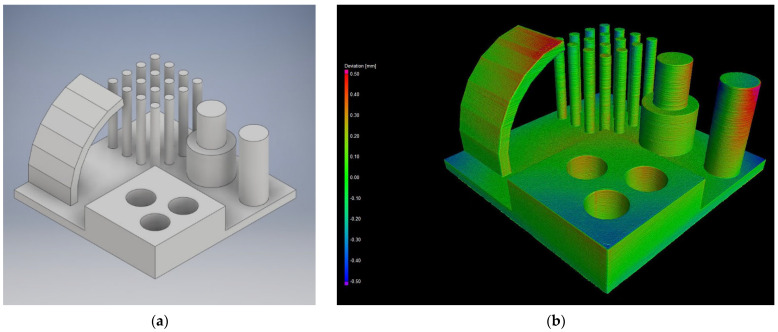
Test artifact for CT analysis. (a) Artifact design in CAD; (b) final measurements dimensional check.
